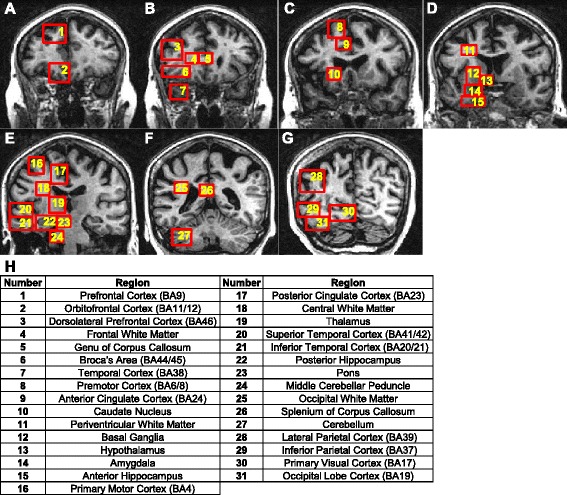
MRI showing the location of brain regions sampled for post-mortem analysis. FLAIR-weighted, coronal MR images from anterior to posterior (a-g) of the LBC1936 participant’s brain. Post-mortem, regions were collected for analysis as highlighted by the numbered boxes. h Numbered boxes correspond to the brain regions labelled in the table, with corresponding Brodmann Area for each cortical region provided
